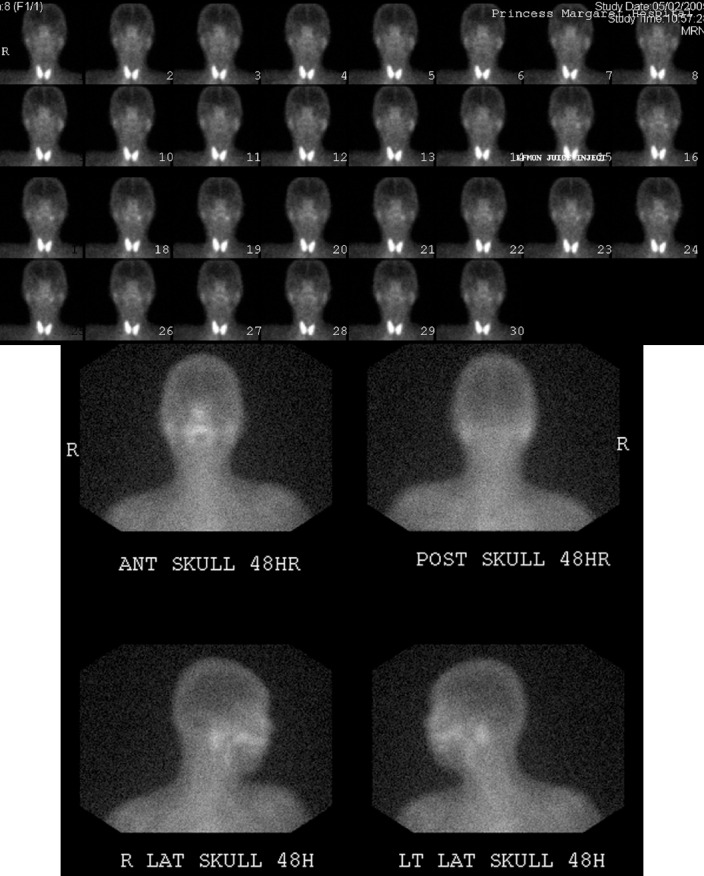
Images of a 34-year-old woman (Patient 10). (A) ^99m^Tc-pertechnetate scintigraphy exhibits decreased accumulation of tracer in left parotid gland. (B) Gallium scan shows marked increased uptake in the bilateral parotid glands and mildly enhanced uptake in the lacrimal glands
